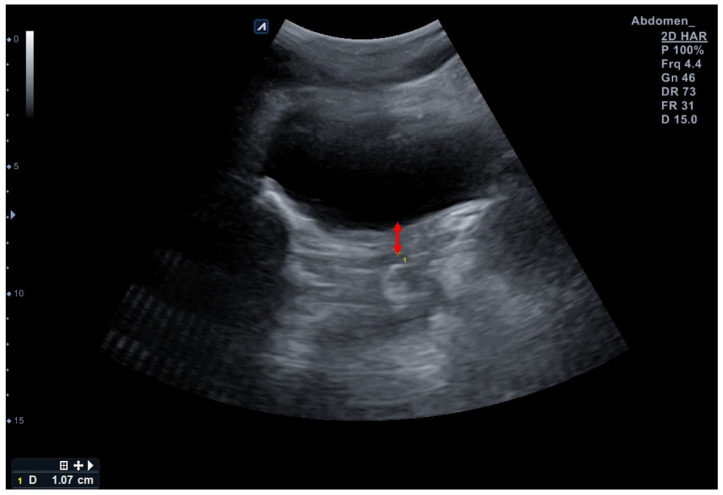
Assessment of bladder base displacement in this study using a transabdominal ultrasound. Red arrow indicates bladder base displacement during contraction.
